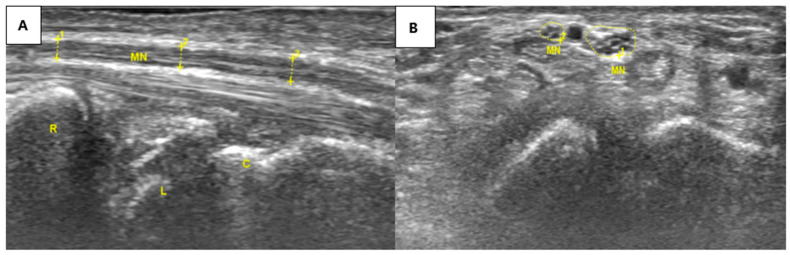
Ultrasound images obtained 10 months after the first treatment session. (A) Long-axis view showing a more uniform median nerve diameter. (B) Short-axis view showing restoration of a distinct fascicular pattern in the bifid median nerve. At this follow-up, the patient reported no numbness or pain in the affected wrist.
